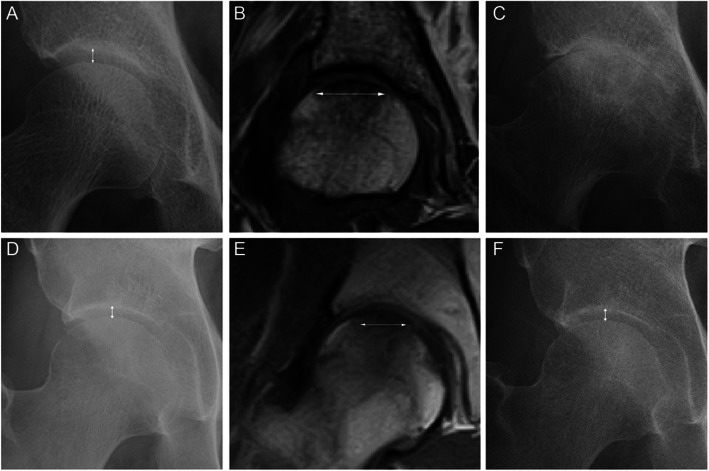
Longitudinal radiological images in patients who underwent total hip arthroplasty and those who did not. a Anteroposterior (AP) radiograph of the hip at the first visit in a 68-year-old female with hip pain. White arrow represents joint space width. b Coronal T1-weighted magnetic resonance image (MRI) of the hip at the same week. White arrow represents length of low-intensity band pattern in the femoral head. c AP radiograph of the hip at the final follow up. Joint space narrowing progressed, and she underwent total hip arthroplasty. d AP radiograph of the hip at the first visit in a 47-year-old male with hip pain. White arrow represents joint space width. e Coronal T1-weighted MRI of the hip at the same week. White arrow represents length of low-intensity band pattern in the femoral head. f AP radiograph of the hip at the final follow-up (3 years after the first visit). Patient demonstrated no progression in joint space narrowing
